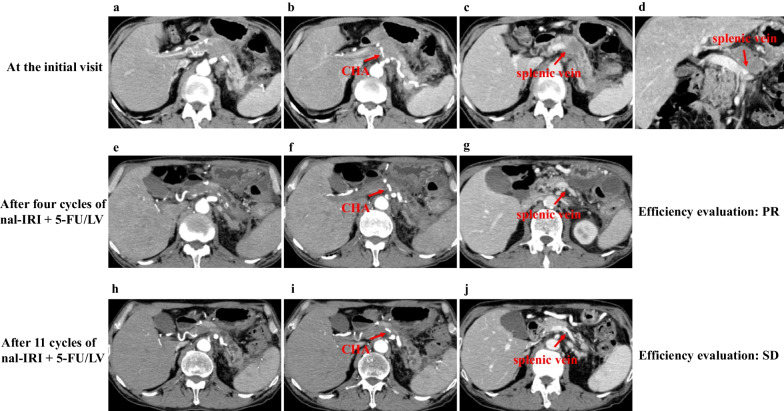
Consecutive CT findings from at baseline to preoperative point. a–d An enhanced mass lesion measuring 33 × 27 mm on the pancreatic body with encasement of the CHA and narrowing of the splenic vein at the initial visit. e–g The tumor shrank to 25 × 10 mm in diameter with the encasement of the CHA and narrowing of the splenic vein after four cycles of liposomal irinotecan + 5-FU/LV chemotherapy regimen administered. h–j The tumor shrank to 18 × 7 mm in diameter with the encasement of the CHA. The narrowing of the splenic vein disappeared after 11 cycles of liposomal irinotecan + 5-FU/LV were administered
